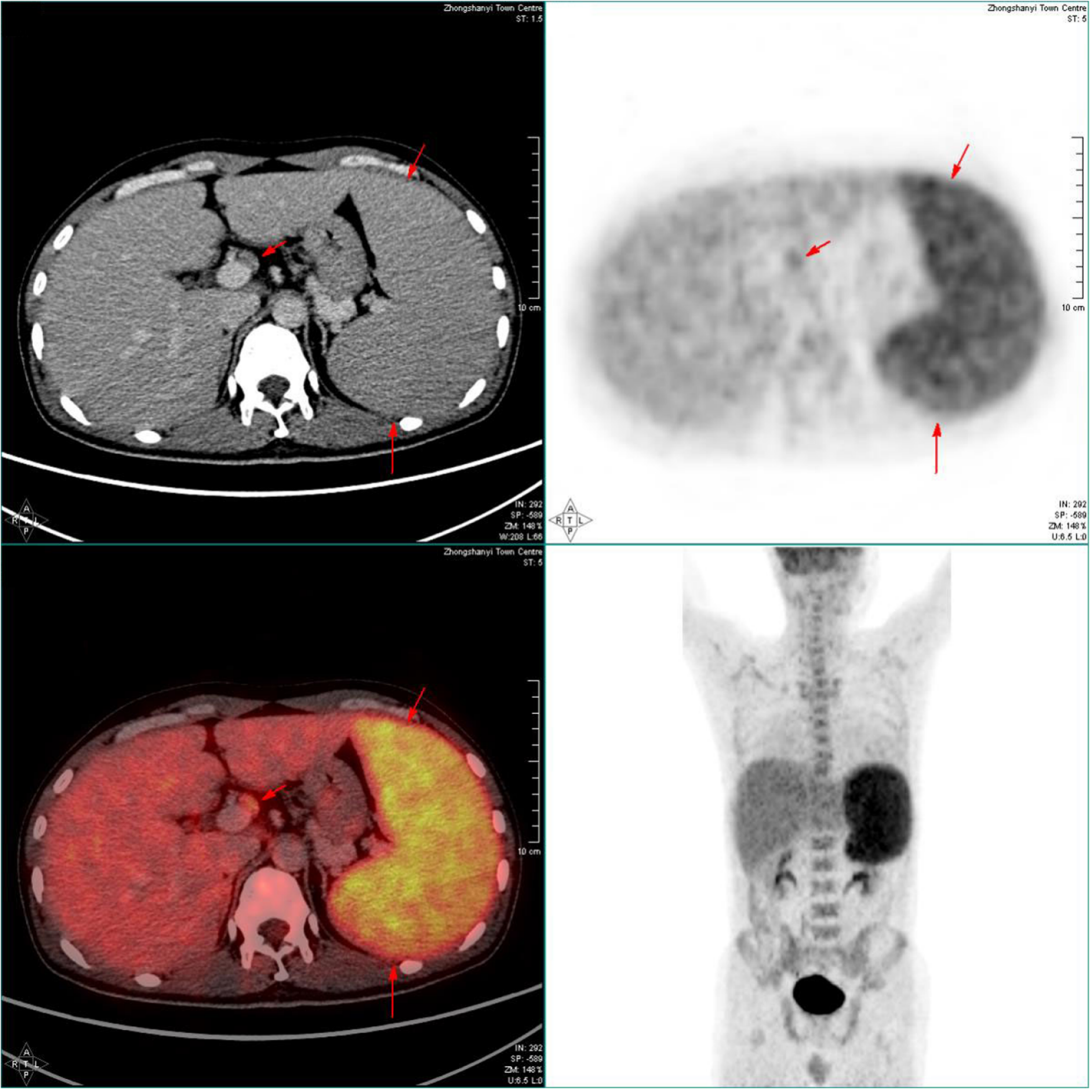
PET-CT images: FDG PET/CT scan showed the patient had marked splenomegaly (long arrow) and hepatic portal lymphadenopathy (short arrow) with increased FDG metabolism. The SUV value of the spleen reached 7.2, and that of the hepatic portal lymph node was 2.8, respectively.
